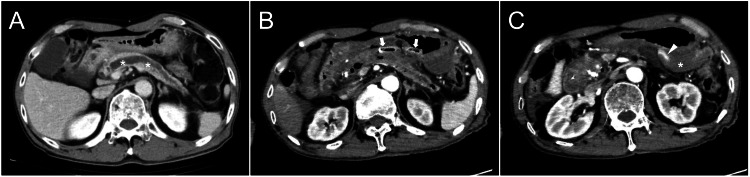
Contrast-enhanced axial computed tomography images obtained before the procedure and after confirmation of bleeding following stent insertion (A) Preprocedural image showing a dilated MPD (*). (B) The stent (arrows) penetrating the gastric wall has migrated from the MPD. Notably, gas density is observed within the MPD, indicating that the stent had indeed been inserted into the duct before migration occurred. (C) Hematoma formation (*) within the omental bursa and contrast medium extravasation (arrowhead) are confirmed. MPD: main pancreatic duct
